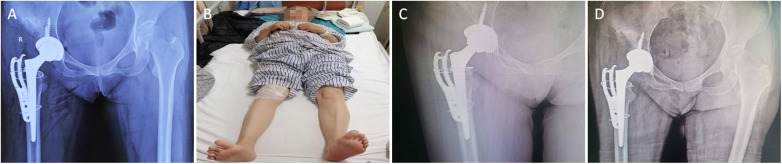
Postoperative x-ray images and external appearance. (A) x-ray images of the patient's right hip THA 1 day postoperatively, showing the femoral fracture line. (B) Both lower limbs are of equal length on the first day after surgery. (C) x-ray examination of the patient's THA 2 months postoperatively, with the proximal femoral fracture line becoming less distinct. (D) The x-ray shows that the proximal femoral fracture has healed, and the prostheses are in good positions, 3 months postoperatively.
